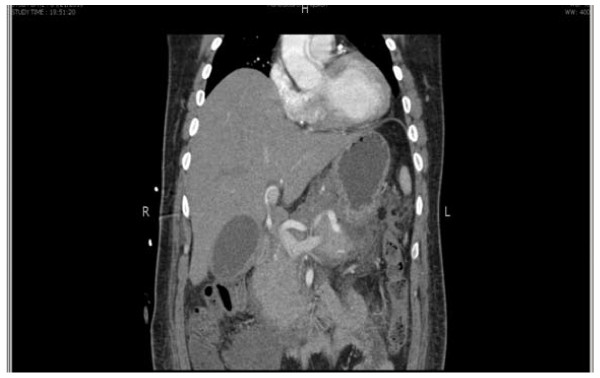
Sagittal view of the computed tomography (CT) scan of our patient showing soft tissue thickening at the gall bladder neck and proximal bile duct region.
